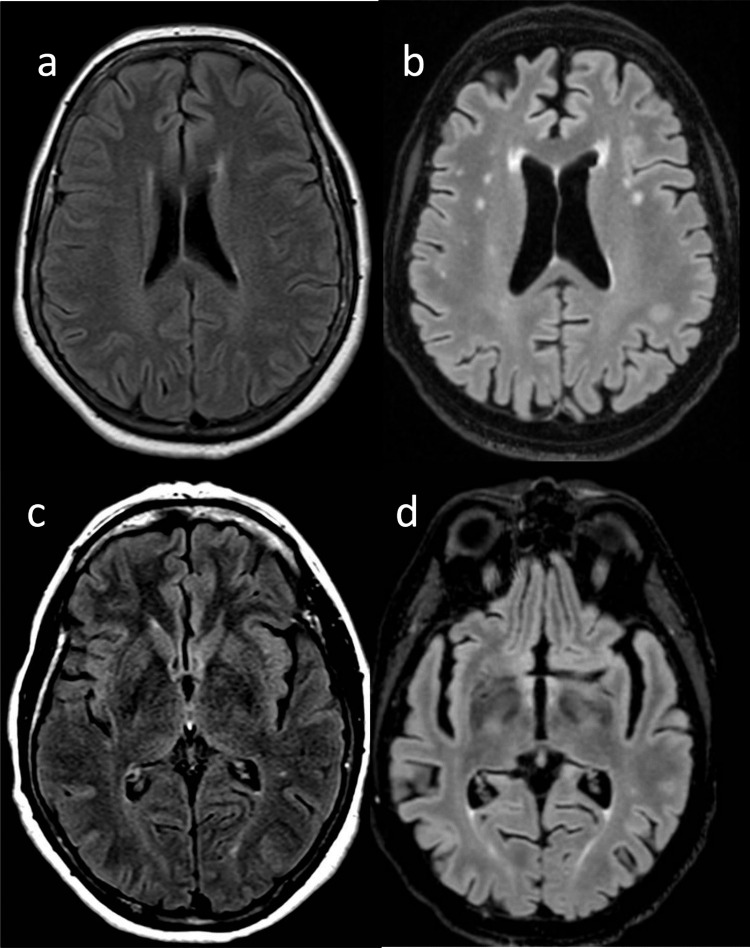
Fluid-attenuated inversion recovery (FLAIR) axial images of brain MRI scans of the proband (a-c: 66 years of age; b-d: 72 years of age). In a and b alterations adjacent to the frontal horns and within the bi-hemispheric white matter are shown, both with progressive increase in time. In d signal alteration of the posterior limb of the internal capsule is shown, which was already present, yet less evident, 8 years before (c)
